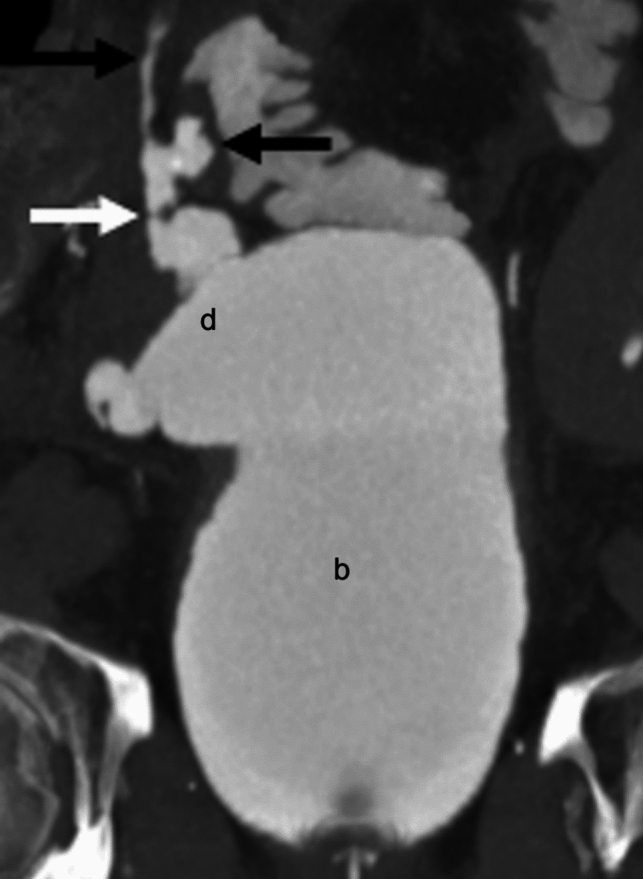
Duodenal stump urine leak following duodenovesical anastomosis. A 58-year-old male with a history of type 1 diabetes mellitus and diabetic nephropathy underwent simultaneous pancreas-kidney transplantation, presenting 10 years after transplantation with elevated pancreatic enzymes. A small fluid collection was seen on contrast-enhanced CT (not shown). CT cystography was performed, which demonstrated contrast outside the bladder (b) and duodenum graft (d) secondary to urine leak (black arrow) near the duodenal stump (white arrow)
